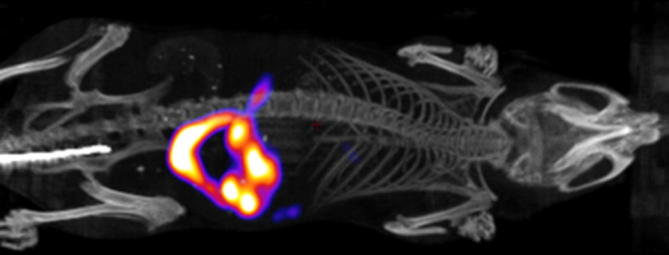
SPECT/CT summation image from 15 to 35 min after injection of [^125^I]10.
